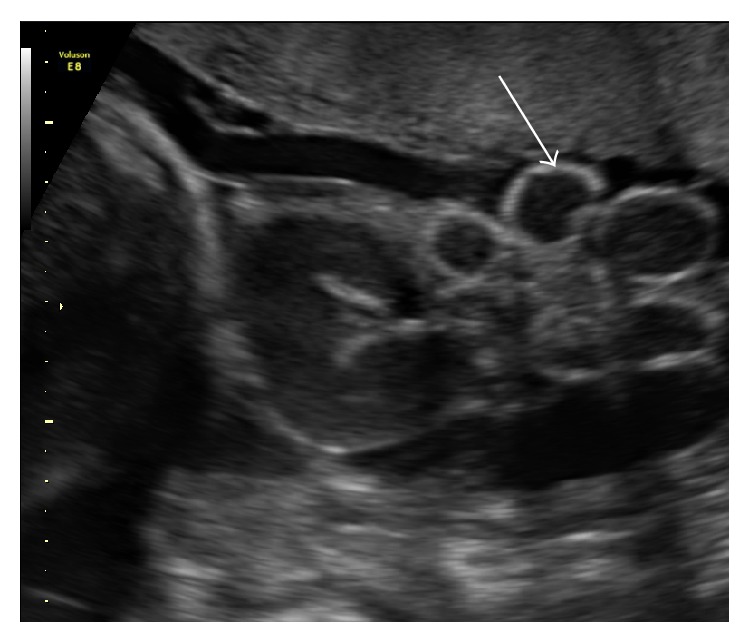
Ultrasound image showing loops of bowel floating free in amniotic fluid in a fetus at 31 weeks of gestation.
